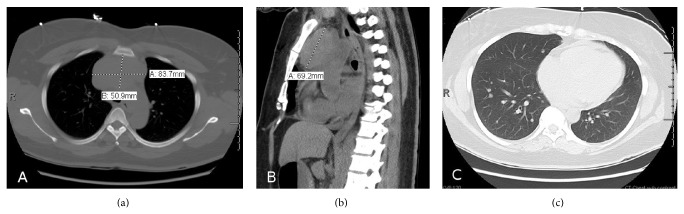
CT scan of the lung showing anterior mediastinal mass measuring 8.4 × 5.1 × 7 cm in anteroposterior (a) and lateral view (b) along with pulmonary nodules (c).
